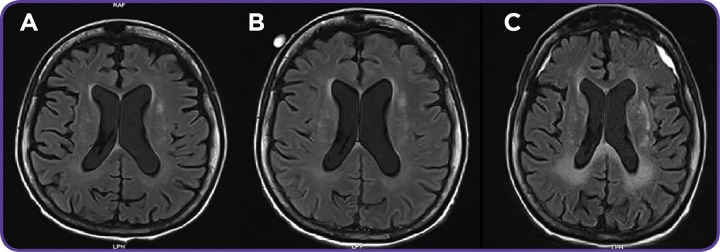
(A) Brain MRI on day 4 posttransplant with generalized atrophy and chronic, microvascular infarcts. (B) Brain MRI on day 11 posttransplant, stable exam compared with prior MRI. (C) Brain MRI on day 41 posttransplant with confluent white matter abnormalities representative of leukoencephalopathy, consistent with viral encephalitis. Also shown are bilateral frontal and posterior parietal subdural hematomas with little mass effect.
